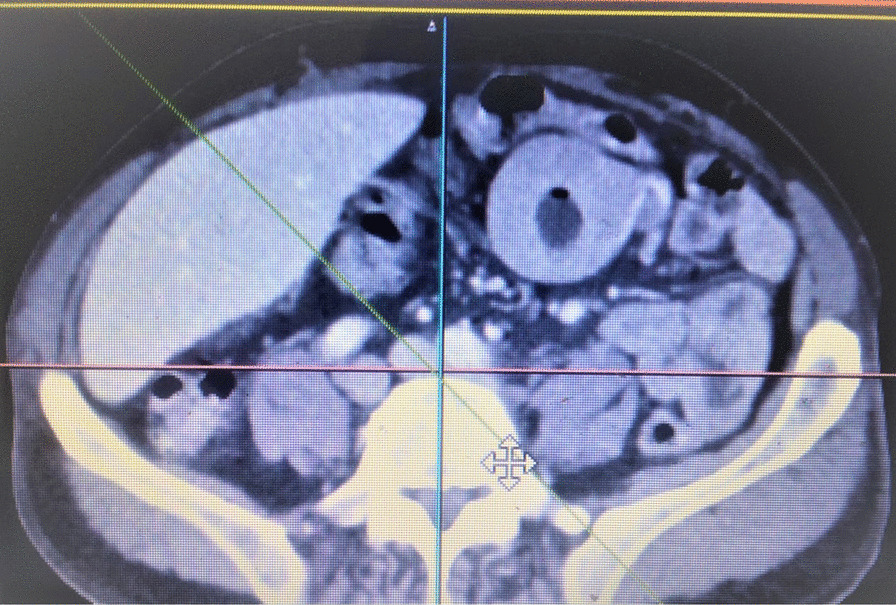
Computed tomography scan at the level of iliac bones showing circumferential mucosal thickening with heterogeneous enhancement suggestive of bowel metastasis
